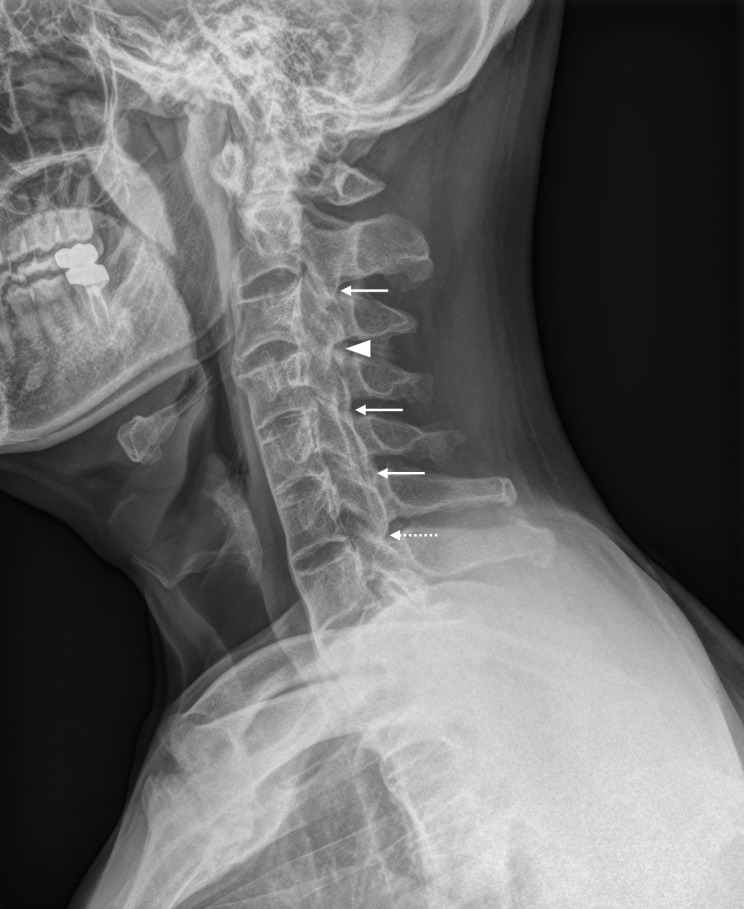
A lateral radiograph of the cervical spine obtained from a 39-year-old ankylosing spondylitis patient with a disease duration of 18 years. At C3–C4 level, cervical facet joint with complete ankylosis is seen (arrowhead, a score of 3 according to de Vlam et al. [14]). Partial ankylosis and joint space narrowing is seen at C2–C3, C4–C5, and C5–C6 (white arrows, a score of 2) and C6–C7 level (dashed arrow, a score of 1), respectively. On the anterior side of the spine, bridging syndesmophtyes are seen
